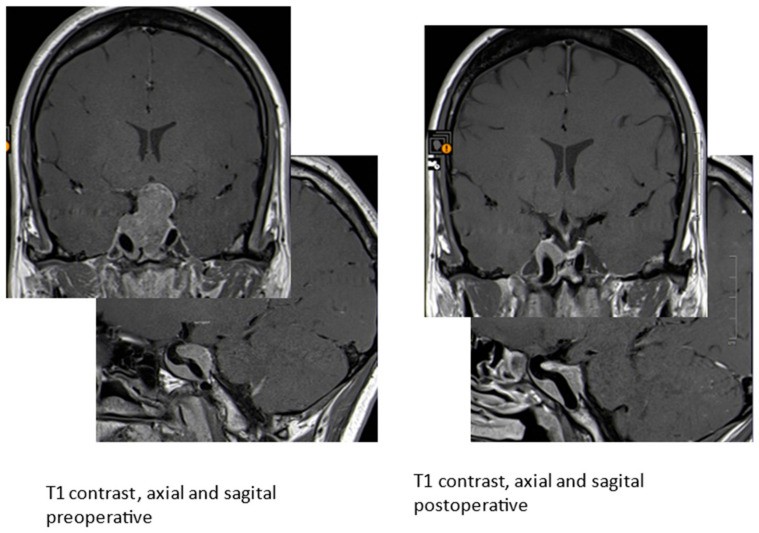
MRI of the sella T1 with contrast media, axial and sagital pre- and postoperative: the tumor encloses the carotid arteries; close to the arteries, remnants had to be left (patient 2).
